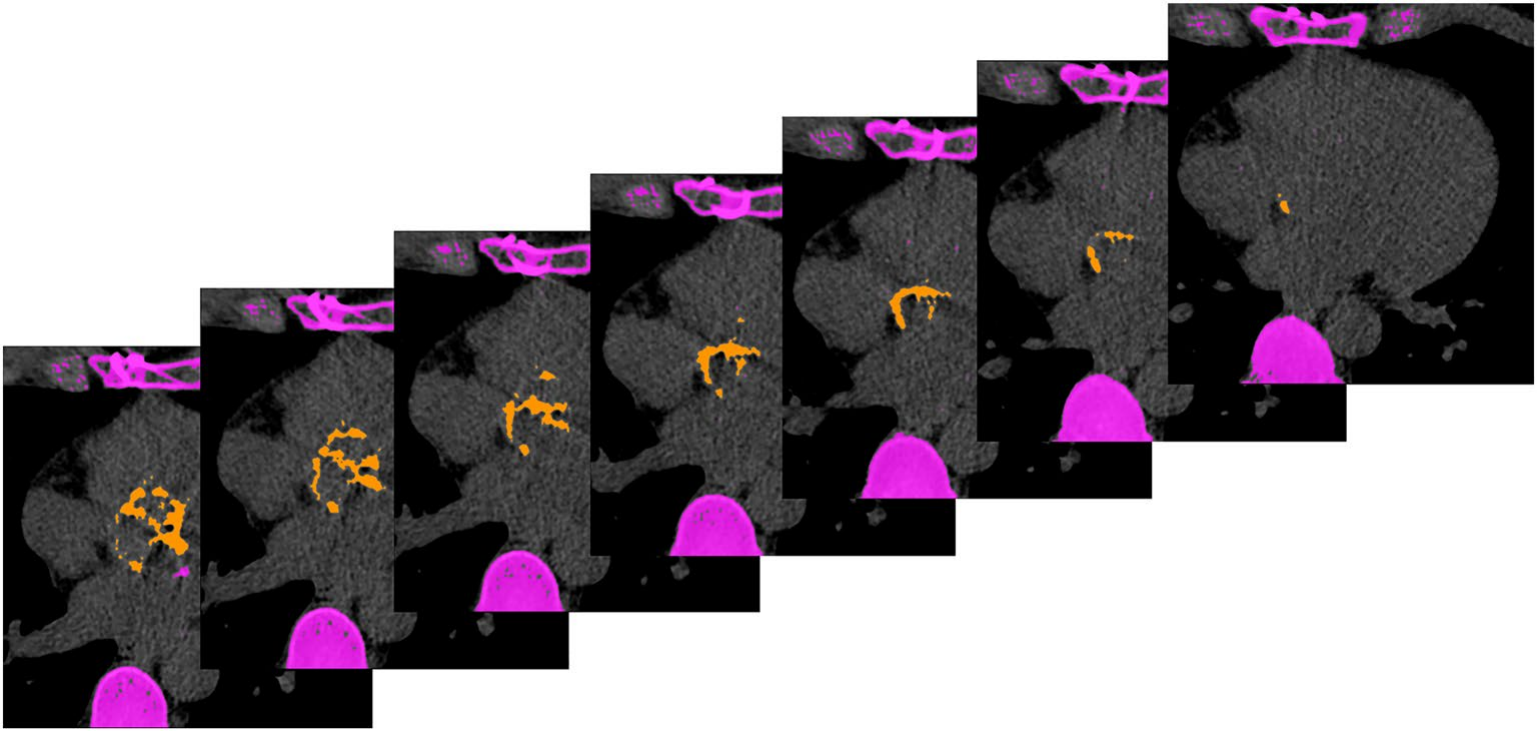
Segmented true non-contrast CT demonstrating severe aortic calcification in a male patient. The aortic valve calcification score (AVCS) was 5808 in this case.
